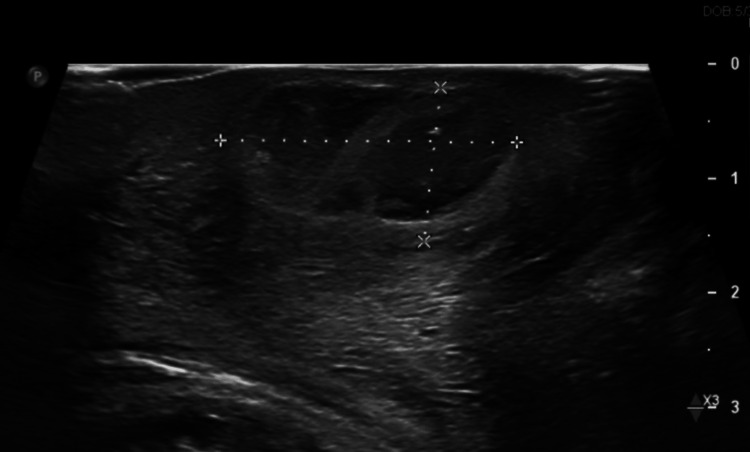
Translabial ultrasound showing a septated periclitoral abscess measuring 2.59 × 1.35 cm between cursors.
